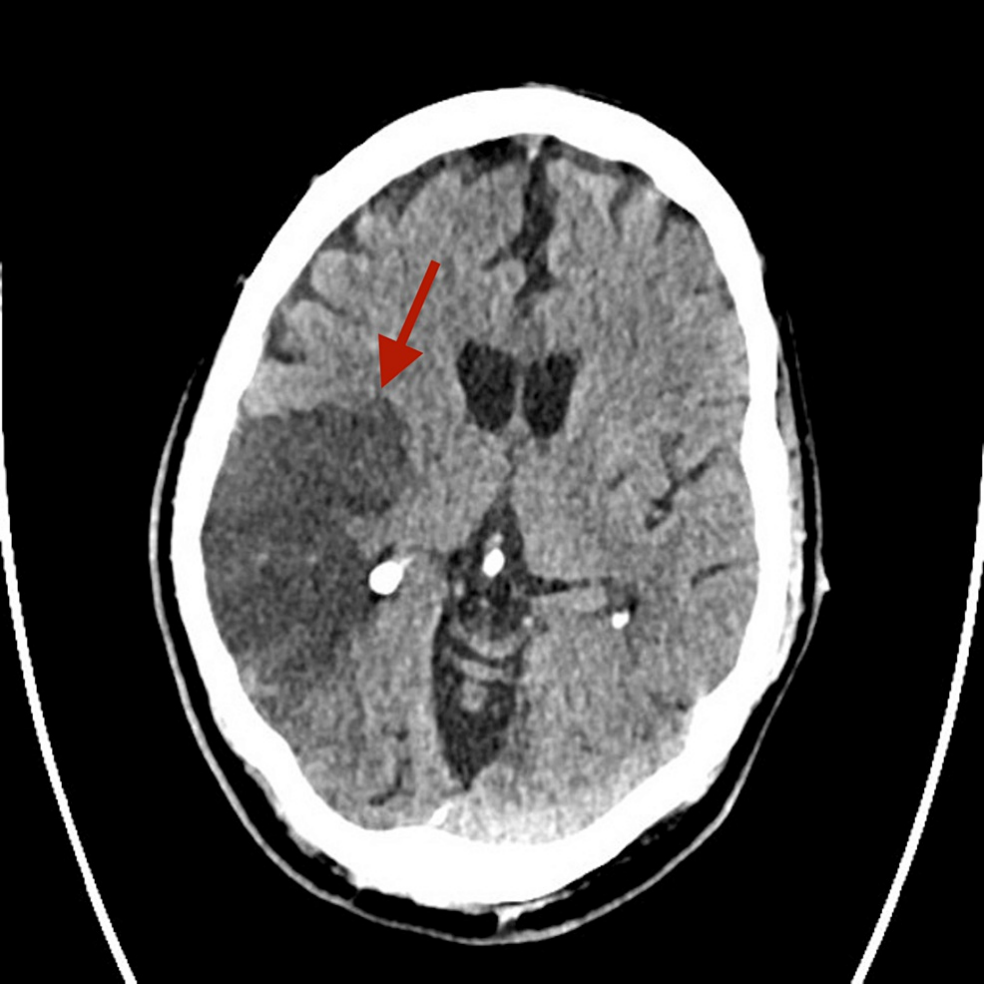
Initial non-contrast CT scan on hospital admission revealing an ischemic stroke in the right middle cerebral artery (MCA) territory (temporo-parietal region).
